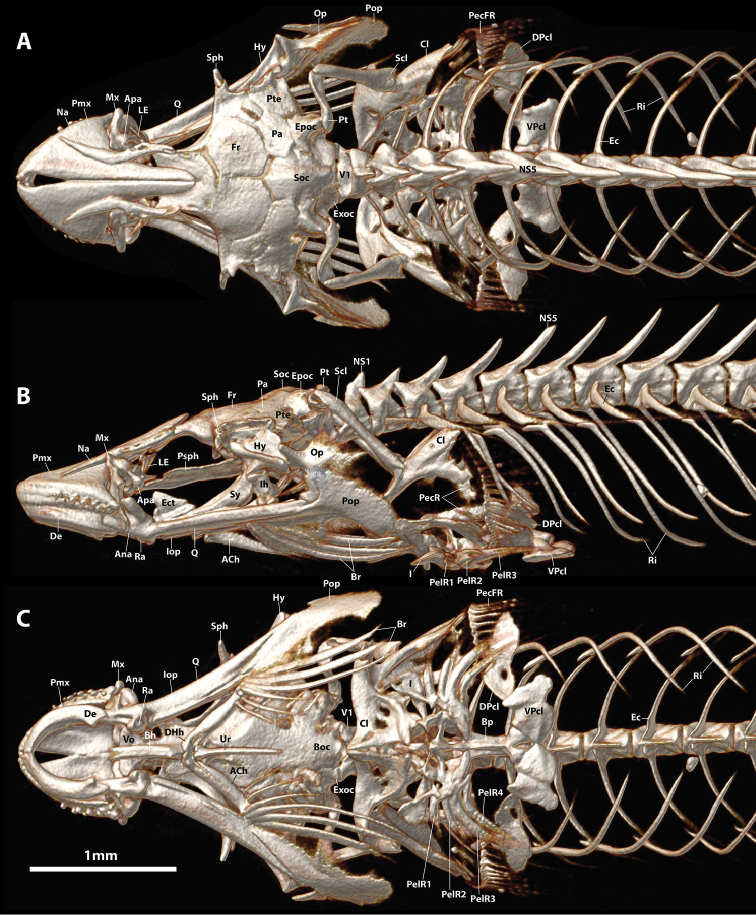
CT scanned anterior skeleton of *Pherallodusindicus*, NSMT-P 114703, 25.0 mm SL. A Dorsal view B Lateral view (left side) C Ventral view. Abbreviations: ACh, anterior ceratohyal; Ana, anguloarticular; Apa, autopalatine; Boc, basioccipital; Bp, basipterygium; Br, branchiostegal rays; Cl, cleithrum; DHh, dorsal hypohyal; DPcl, dorsal postcleithrum; Ec, epicentral; Ect, ectopterygoid; Epoc, epiotic; Exoc, exoccipital; Fr, frontal; Hy, hyomandibular; I, pelvic-fin spine; Iop, interopercle; LE, lateral ethmoid; Na, nasal; NS1, 5, neural spine of vertebral centrum 1, 5; Op, opercle; Pa, parietal; PecR, pectoral radial; PecFR, pectoral-fin ray; PelFR1-4, pelvic-fin ray 1–4; Pop, preopercle; Pro, prootic; Psph, parasphenoid; Pt, posttemporal; Pte, pterotic; Q, quadrate; Ra, retroarticular; Ri, rib; Scl, supracleithrum; Soc, supraoccipital; Sph, sphenotic; Ur, urohyal; V1, vertebral centrum 1; Vo, vomer; VPcl, ventral postcleithrum.
